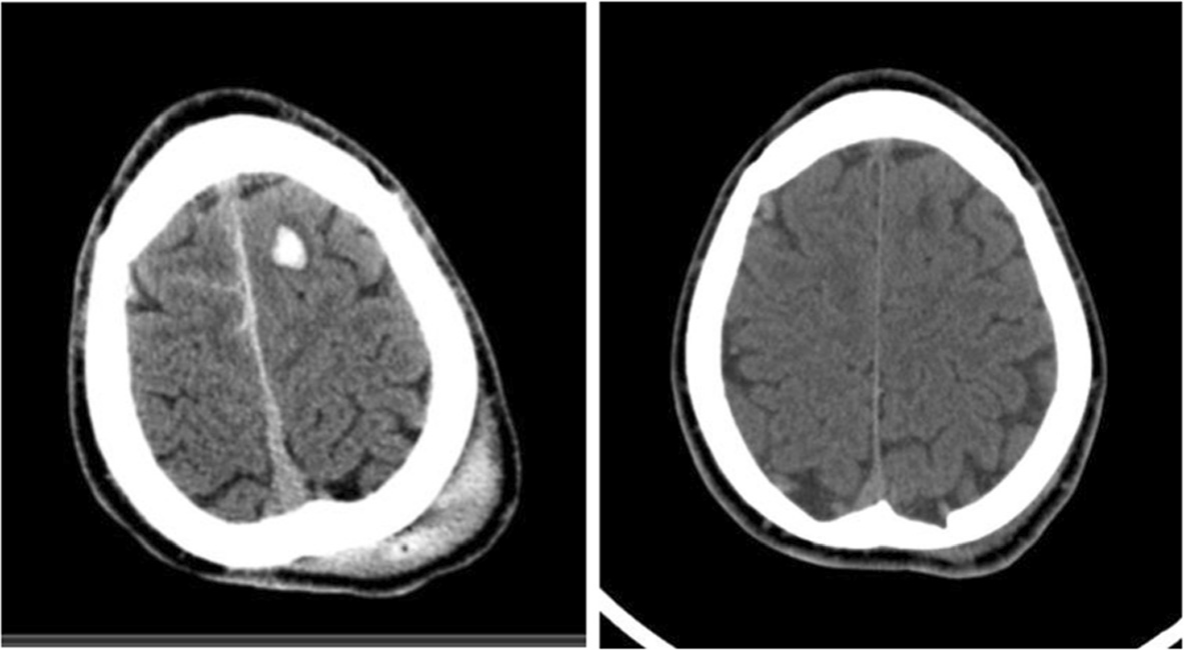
False negative subject. A 20-year-old with GCS 14 and unclear episode of loss of consciousness. The patient would be classed as low-risk mild traumatic brain injury and had a S100B of 0.09 μg/L. CT shows a small contusion and extracranial soft tissue swelling. The contusion subsided on follow-up CT after 25 days. The patient did not need any intervention or treatment and was discharged from the inpatient unit with a good neurological outcome
